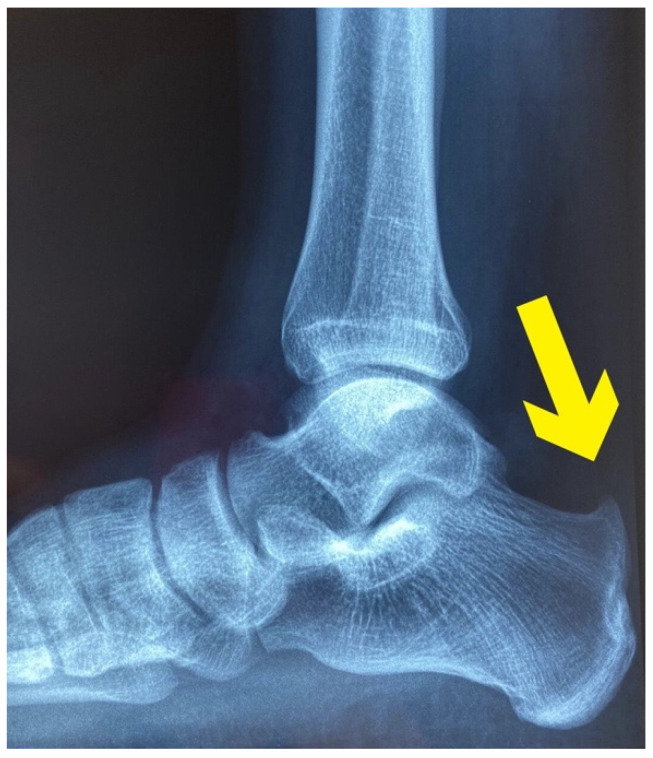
X-rays: The yellow arrow indicates an abnormal exostosis on the posterior superior aspect of the calcaneal bone.
